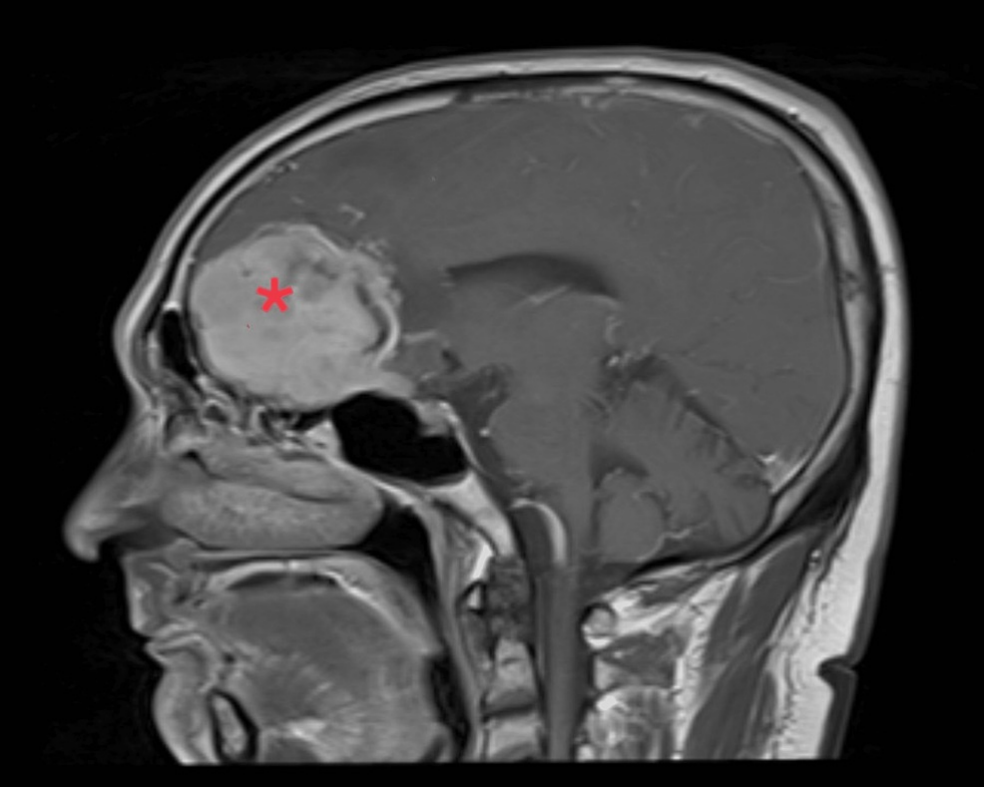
MRI (sagittal view) showing the anterior skull base meningioma (red asterisk) causing mass effect (right more than left), left midline shift, and contralateral early hydrocephalus.
